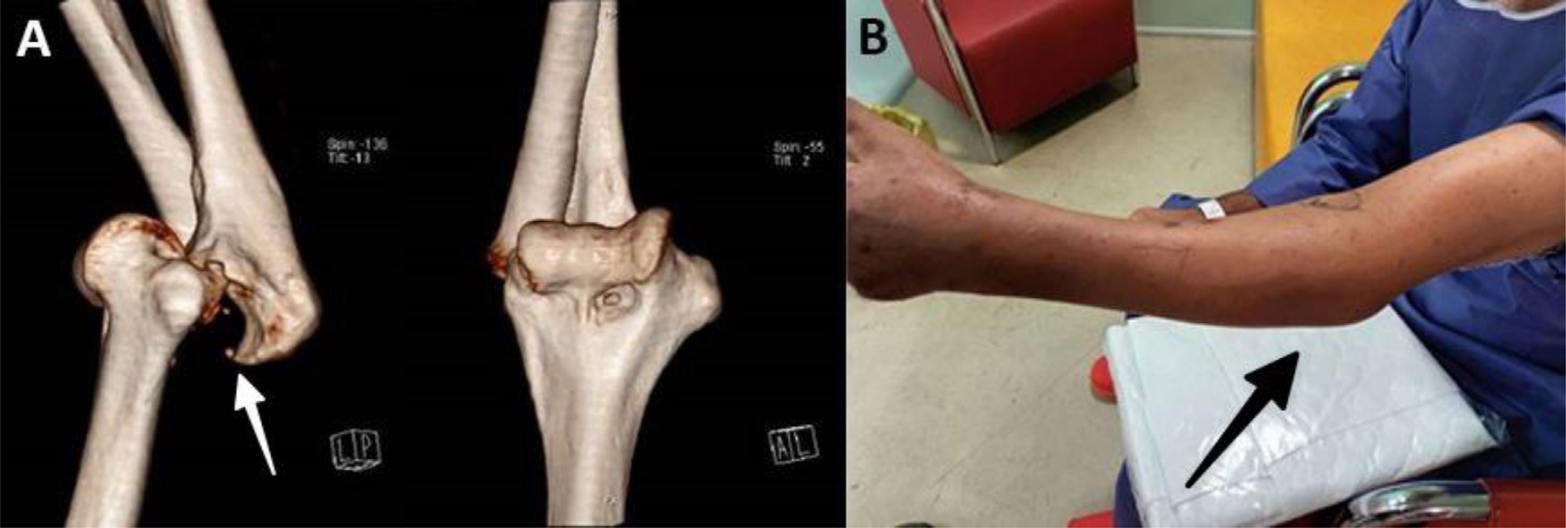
Documentation of neglected posterior elbow dislocation. (A) 3D volume-rendered CT reconstruction in lateral and AP views showing posterior displacement of the ulna relative to the distal humerus (white arrow) with loss of articulation between the trochlea and sigmoid notch. Note the disruption of the ulnohumeral line and dislocation of the radial head. (B) Clinical photograph demonstrating fixed flexion deformity and abnormal contour of the elbow joint (black arrow) with limited range of motion.
